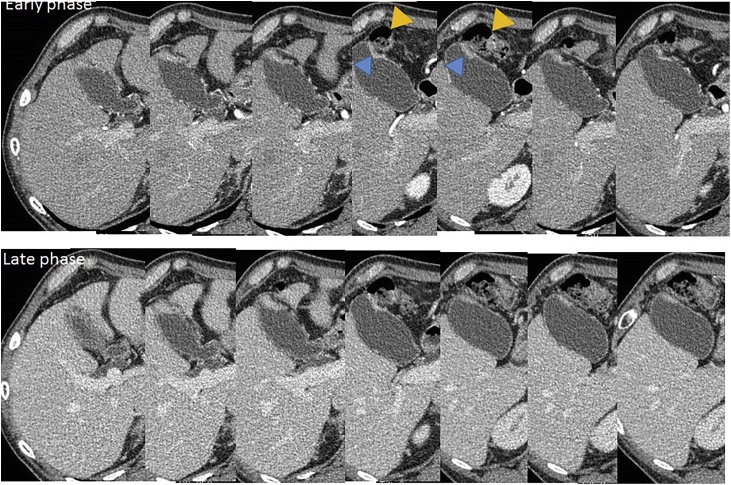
Computed Tomography Images. (For interpretation of the references to colour in this figure legend, the reader is referred to the web version of this article.) Enhanced mass was shown at the tail of the gallbladder (blue triangle). Tumor was adjacent to the transverse colon (yellow triangle).
